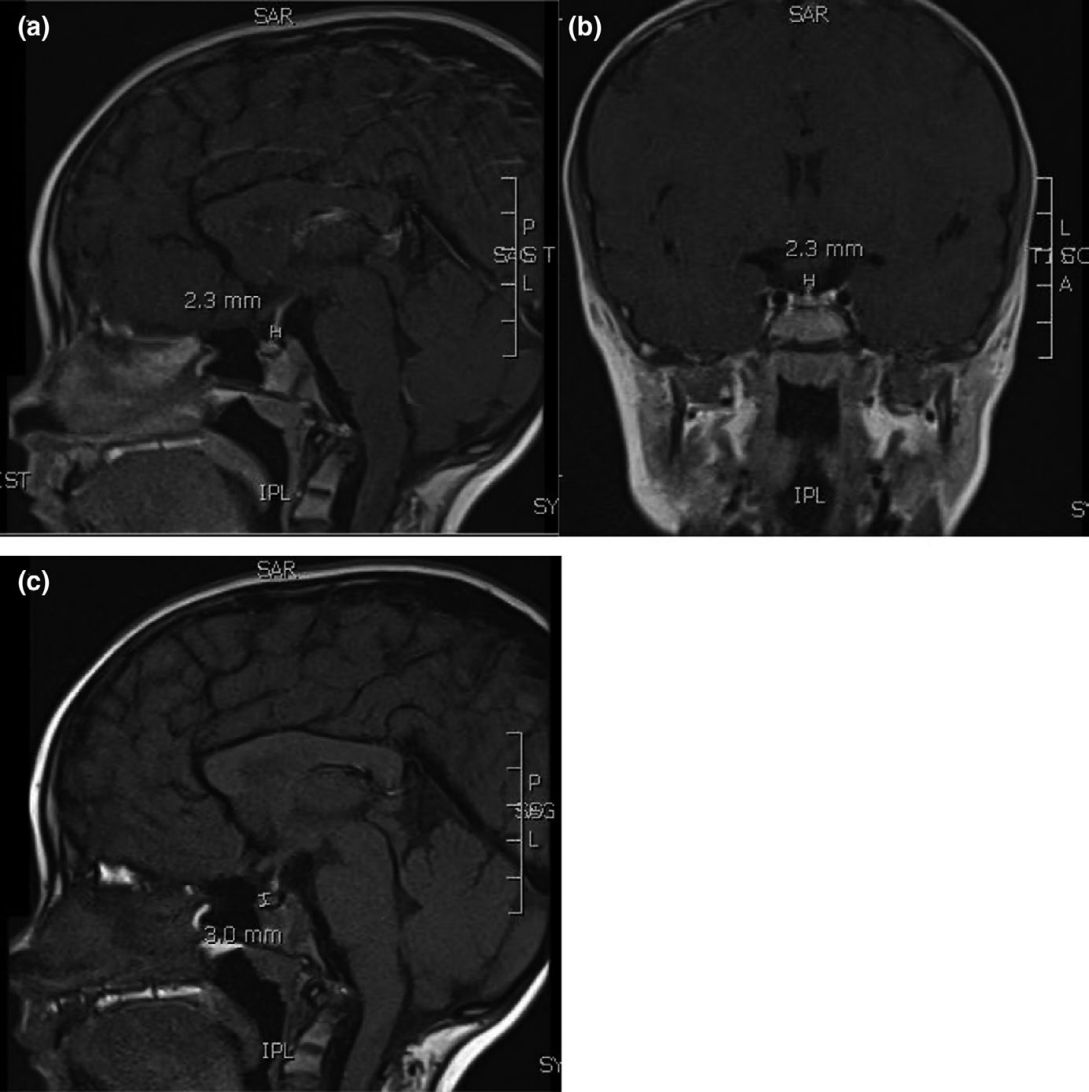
Magnetic resonance imaging of Sister 2. MRI of brain and sella was performed with and without gadolinium‐based intravenous contrast. Coronal and sagittal T1‐weighted images show ectopic posterior pituitary (EPP) and anterior pituitary hypoplasia. (A and B) There is a focal T1 hyperintense thickening of the infundibulum measuring 2.3 mm with a normal posterior pituitary bright spot not seen within the sella most consistent with an EPP. (C) The anterior pituitary gland is small measuring 3 mm in height
